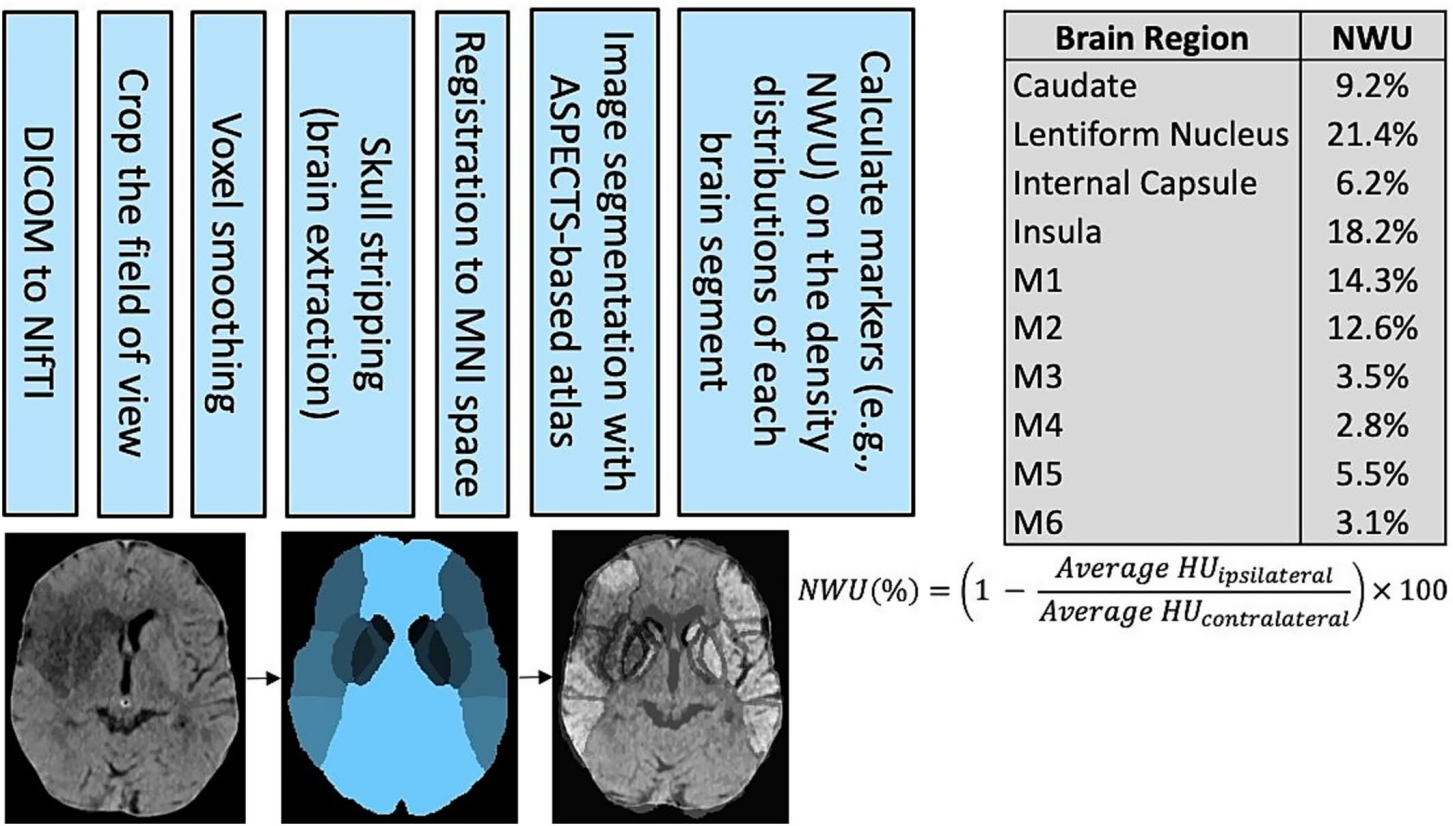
The algorithm for automated net water uptake calculation includes pre-processing, segmentation, and density calculations. On the right, the example results demonstrate how NWU quantifies the degree of injury in each stroke region. DICOM, Digital Imaging and Communications in Medicine; NIfTI, Neuroimaging Informatics Technology Initiative; MNI, Montreal Neurological Institute; ASPECTS, Alberta Stroke Program Early CT Score; NWU, net water uptake; and HU, Hounsfield Units.
